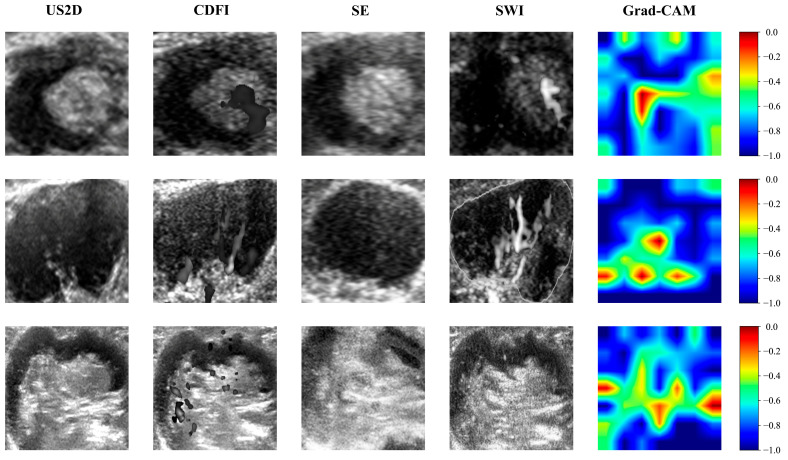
Grad-CAM activation maps of ultrasound images for DLBCL prognostic prediction.
